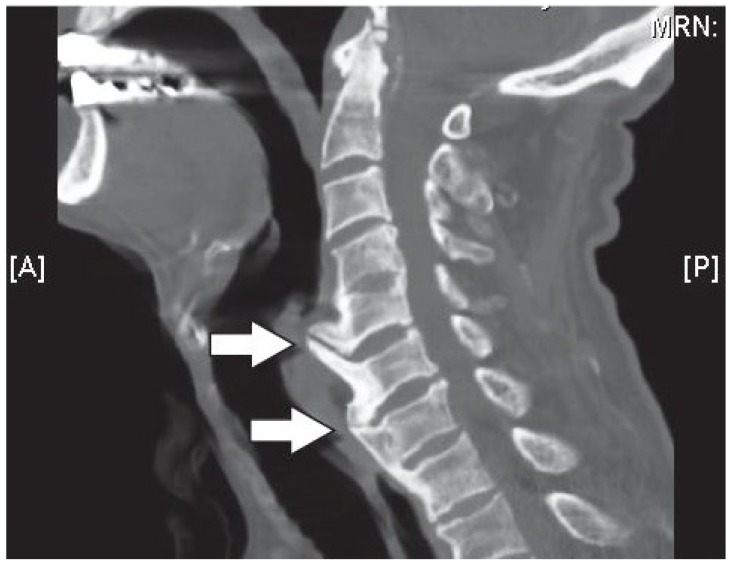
Sagittal computerized axial tomography (CT scan) image of the cervical spine showing large anterior osteophytes (indicted by the arrows) extending from C5 to C7, which affect the intervertebral disc space [95].
